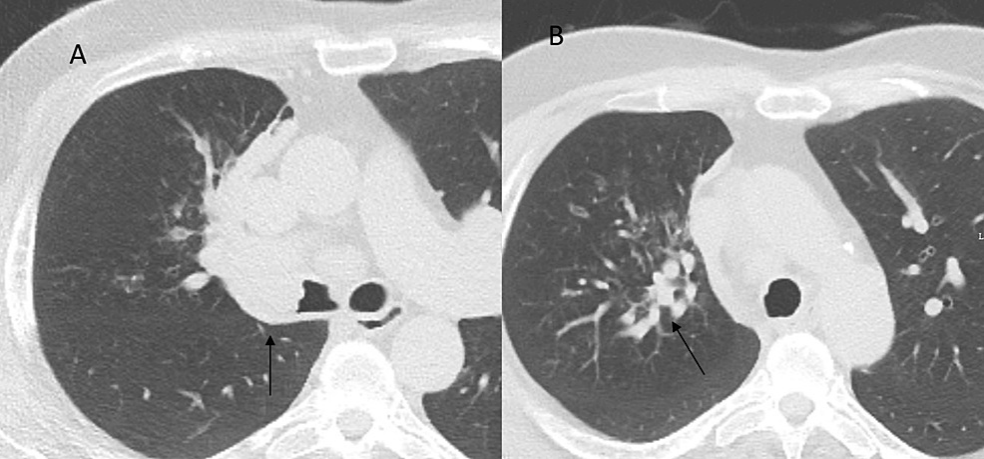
Chest CT showing an endobronchial mass (A) and bronchocele resulting from mucus plugging distal to the tumor obstruction in segmental and subsegmental bronchi of the right upper lobe (B). We clearly see enlarged and filled bronchi in the right upper lobe compared to the contralateral side.
